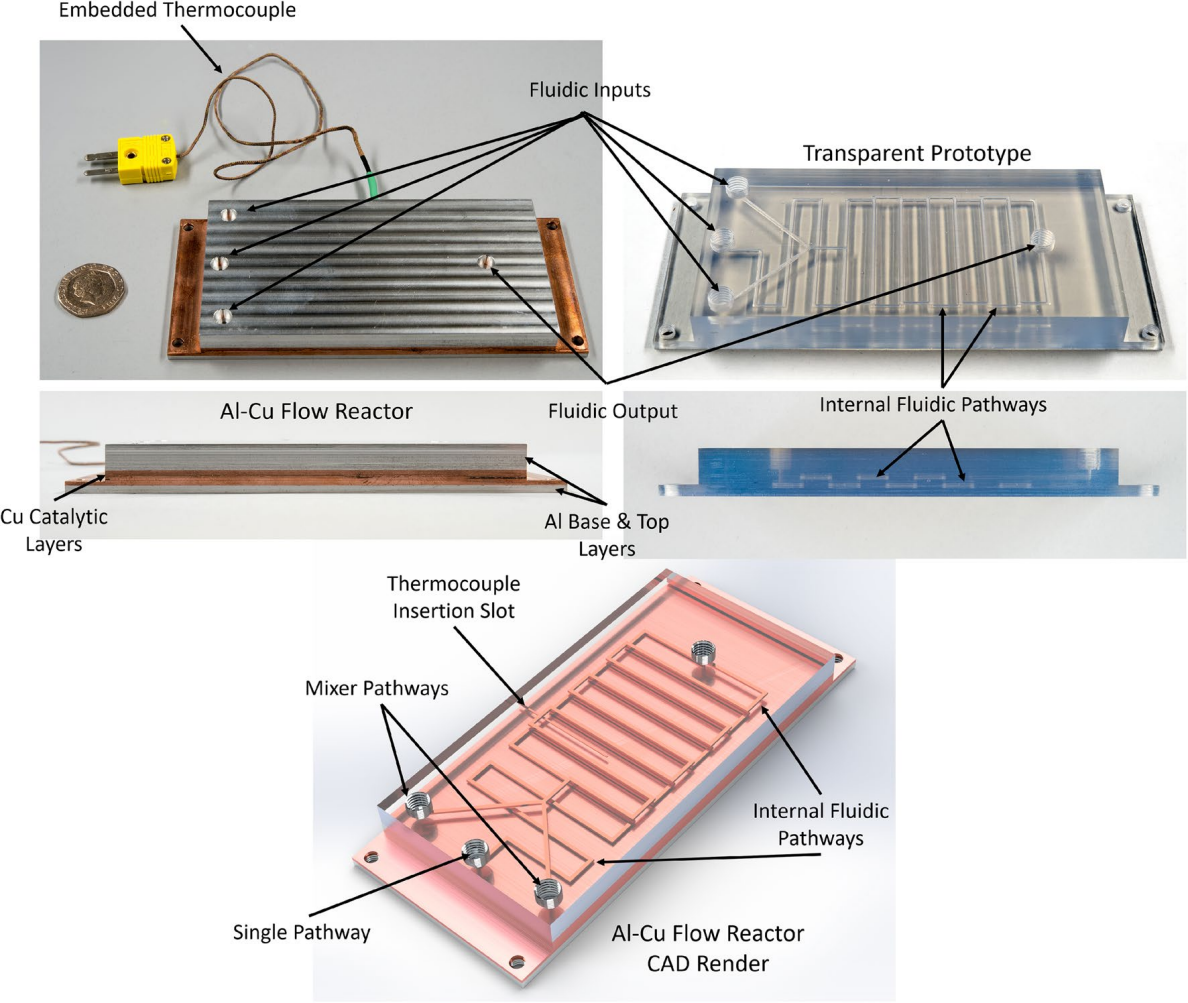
Al-Cu Flow Reactor manufactured via Ultrasonic Additive Manufacturing (UAM), featuring complex internal channel structures, embedded thermocouple and the catalytic reaction chamber. For visualisation of the internal fluidic pathway, a transparent prototype manufactured using Stereolithography is also shown.
